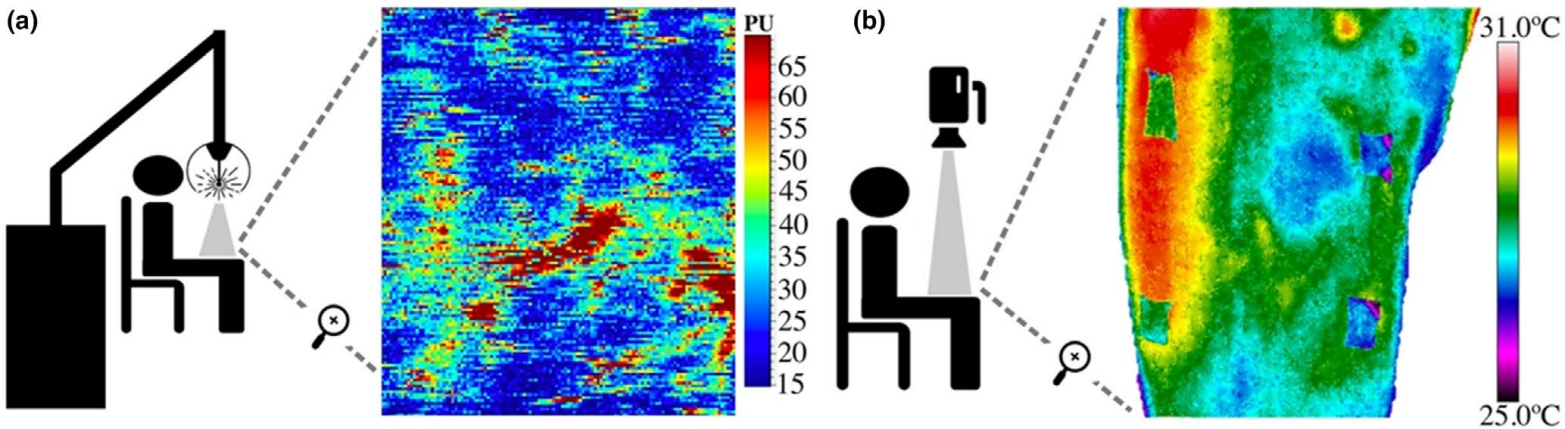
Laser Doppler (a) and IRT camera (b) placements and images.
